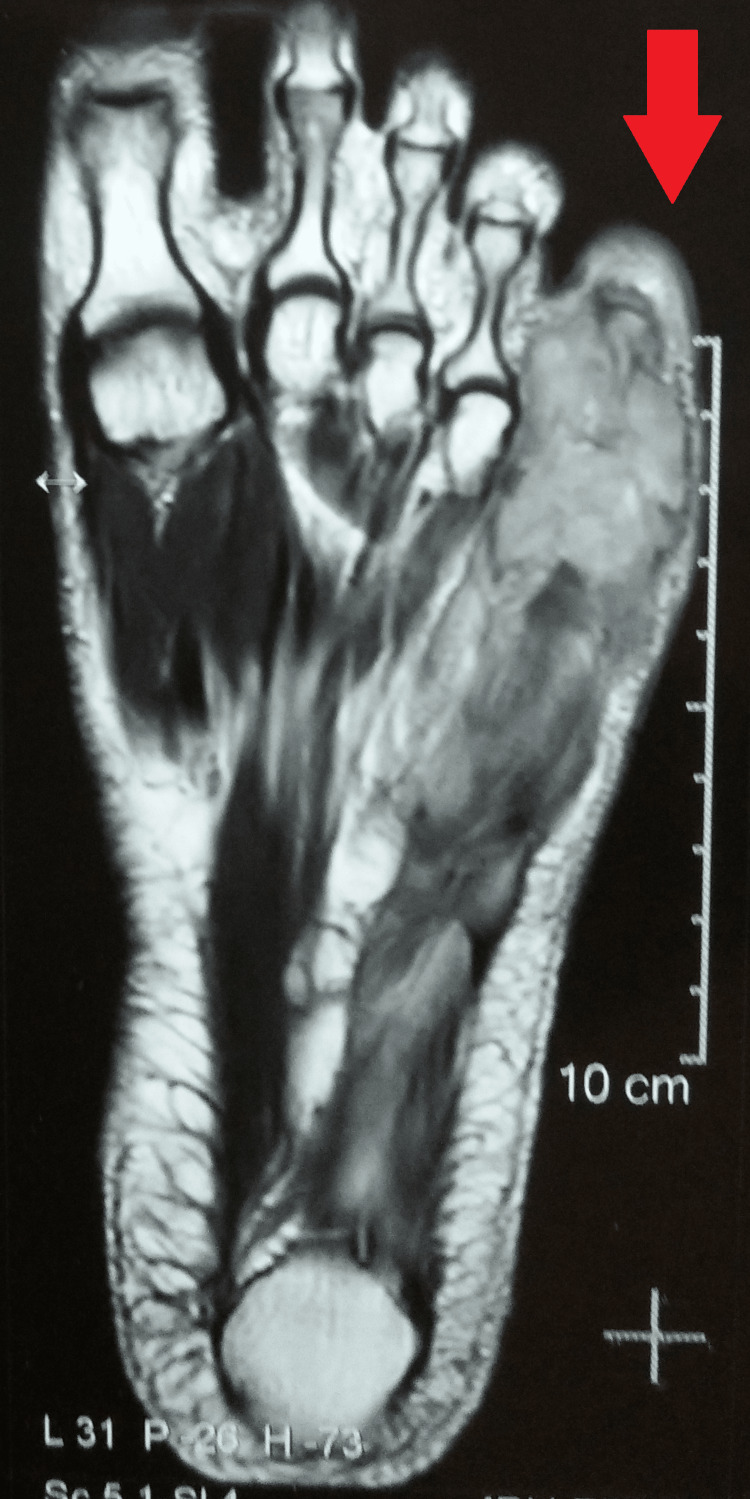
A MRI of the left foot showing the involvement of the distal fifth metatarsal and proximal phalanx MRI: magnetic resonance imaging
